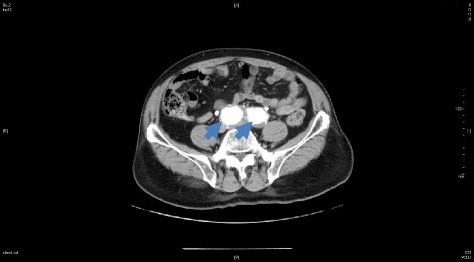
Preoperative CT images of the iliac aneurysm: Case 1.
